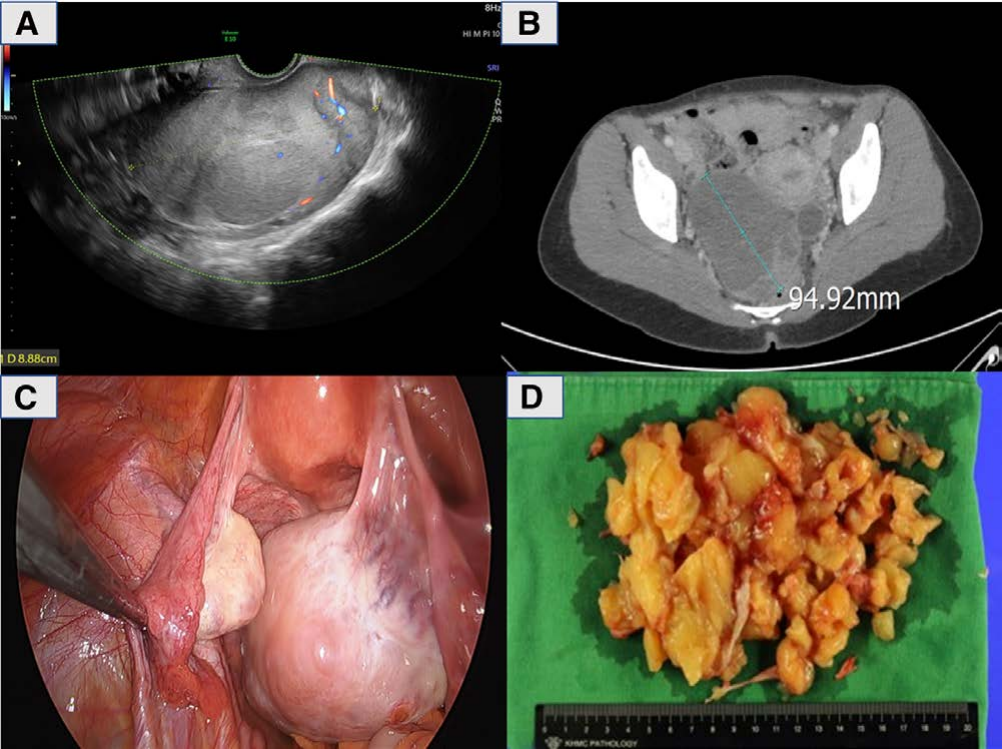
Findings of right ovrian mass. (A) Ultrasound showed about 9cm right ovarian tumor. (B) Abdominal computed tomography (CT) revealed a large poorly enhancing soft tissue mass at the right adnexa (about 9.4 × 7.0 × 6.8 cm sized). AJCC. (C) Laparoscopic surgery finding. (D) Gross examination of the tumor was performed in the fragmented tissue state, measuring 15 cm in aggregation. The tumor was yellow-tan, soft mass and showed abundant myxoid area.
